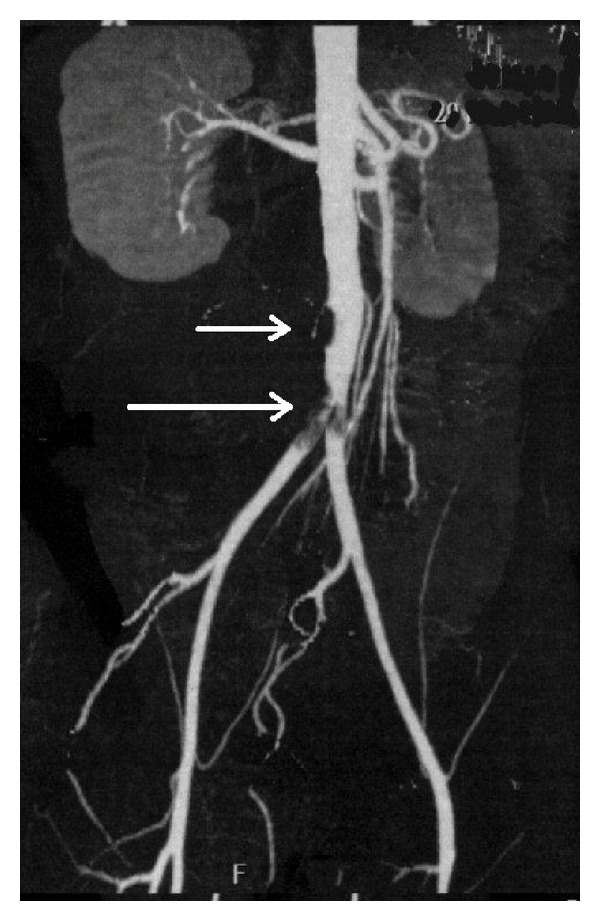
CT angiography showing the presence of an intraluminal aortic (short arrow) and iliac (long arrow) saddle embolus.
